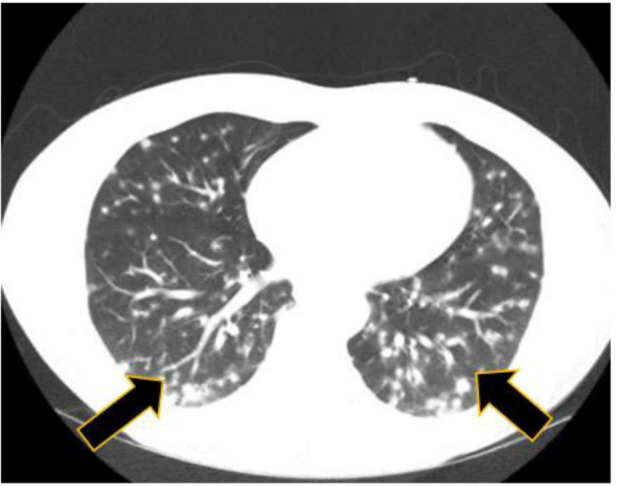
Patient 1 CT scan chest with evidence of diffuse granulomatous disease
